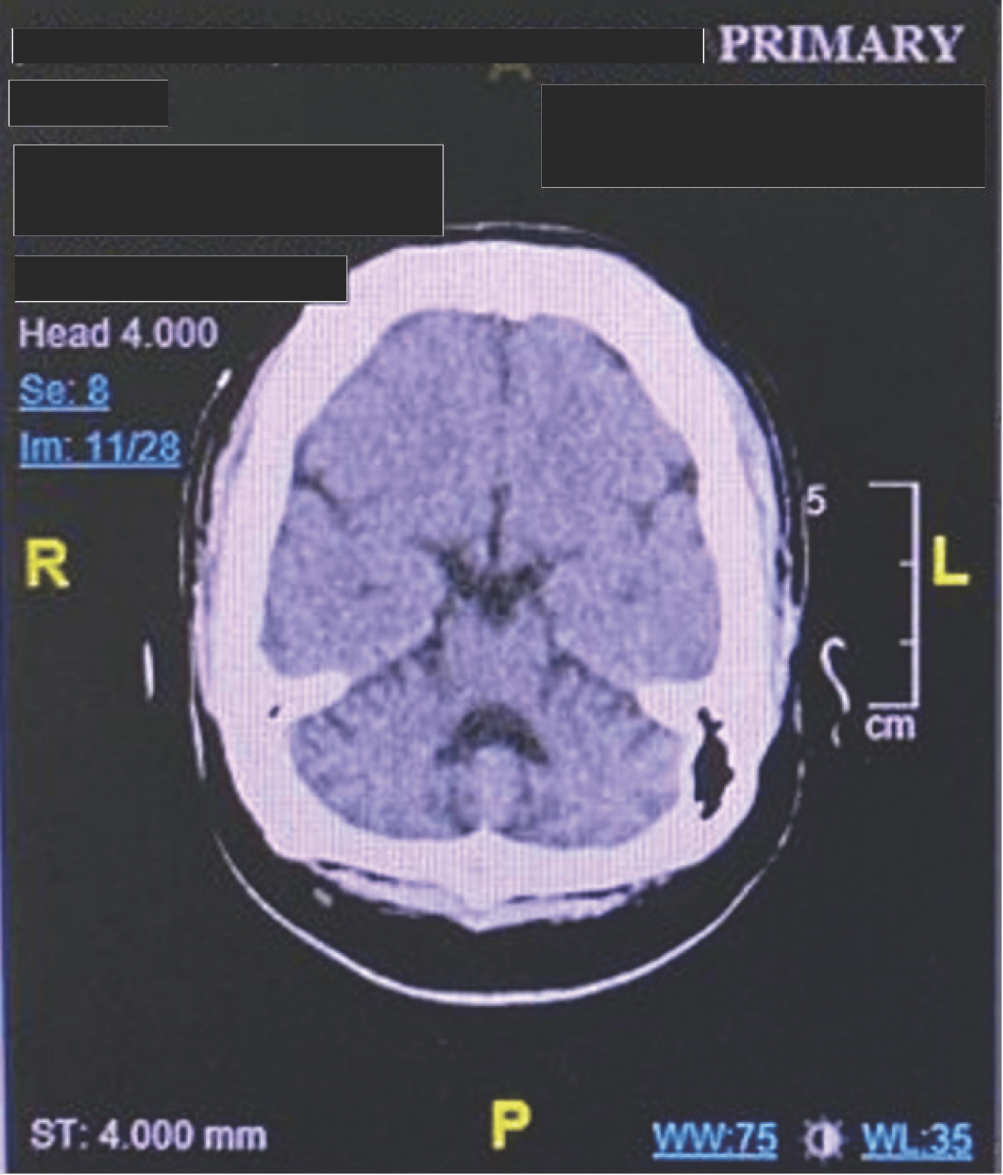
Non-contrast CT brain demonstrating preserved gray-white matter differentiation Contrast-enhanced imaging was not performed; therefore, leptomeningeal enhancement was not directly assessed CT: computed tomography
